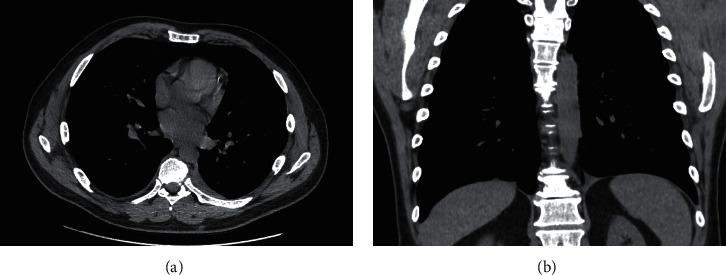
Repeat CT demonstrating resolution of hydropneumothoraces in the (a) axial and (b) coronal views.
